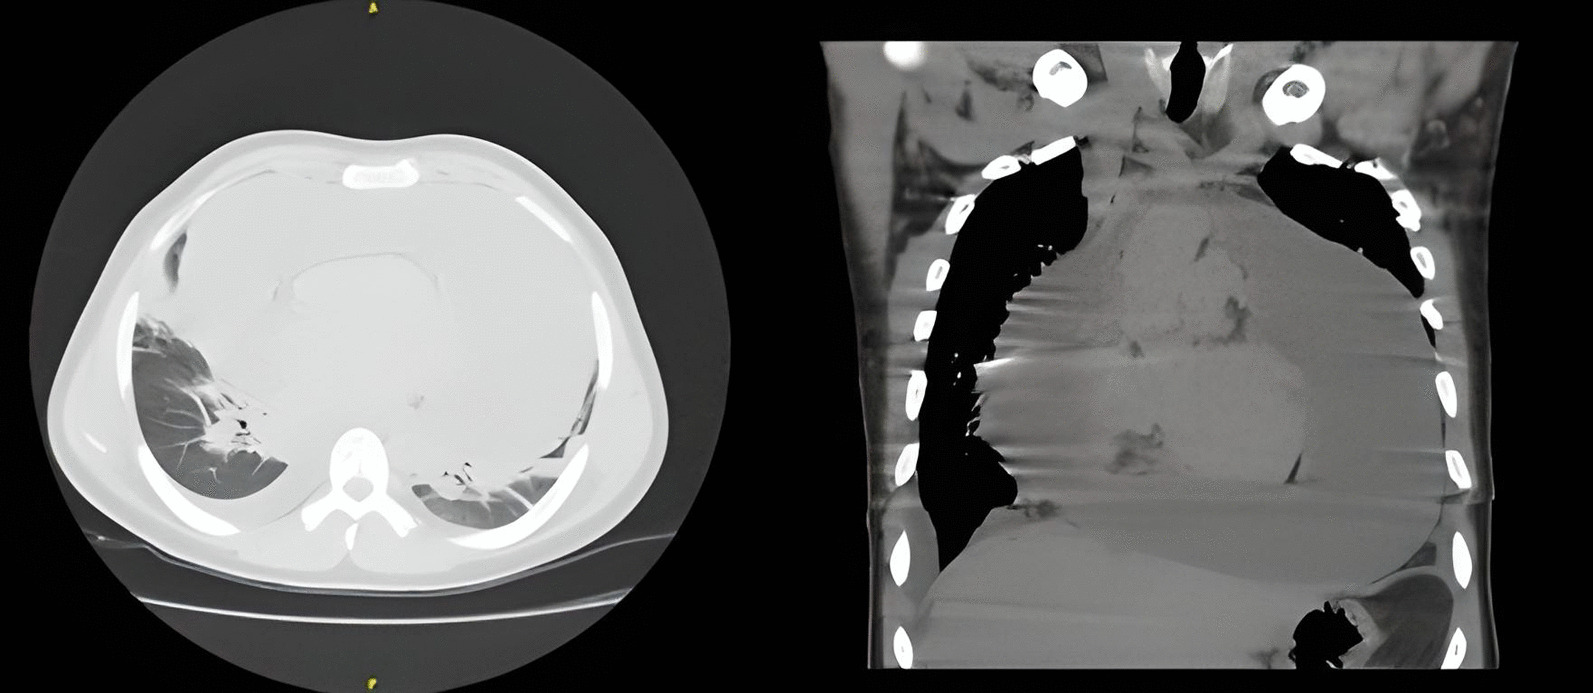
Chest computed tomography showing a massive
